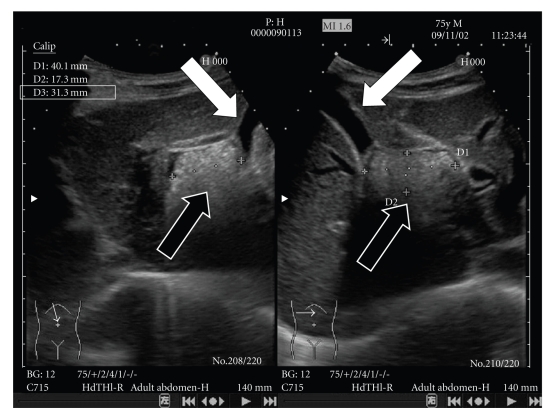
Main gallbladder (white arrow) and cystic structure with multiple stones and debris (black arrow) were confirmed by ultrasonography.
